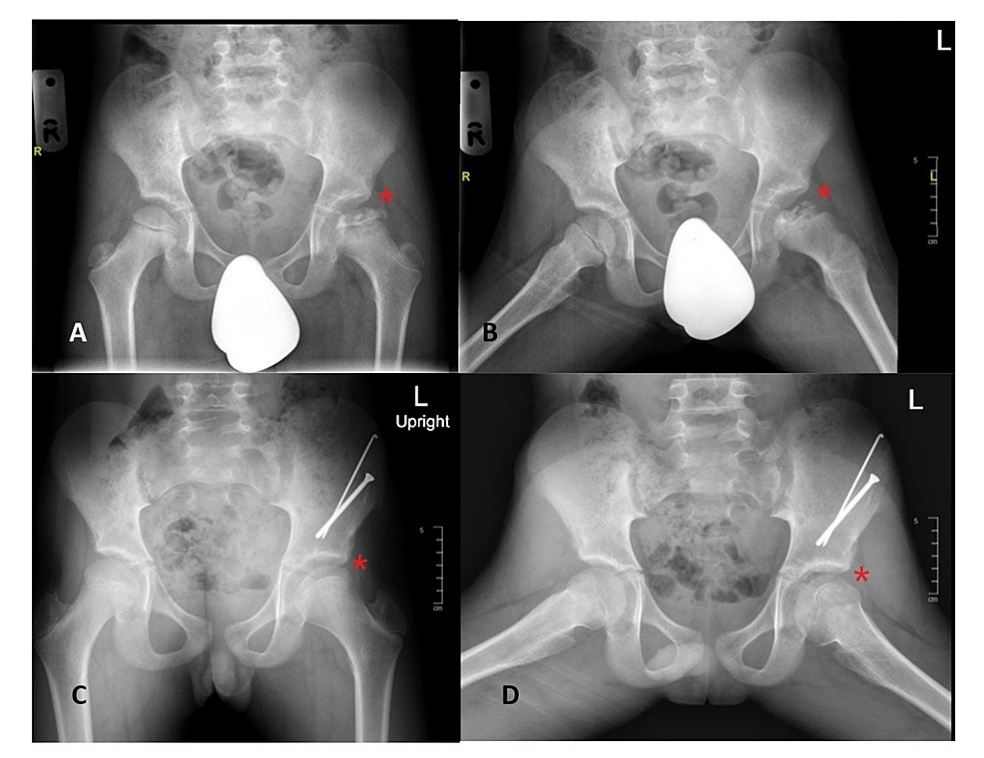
Example pf pelvic x-rays of one patient with Legg-Calvé-Perthes disease older than eight years before (A,B) and after (C,D) surgical treatment
